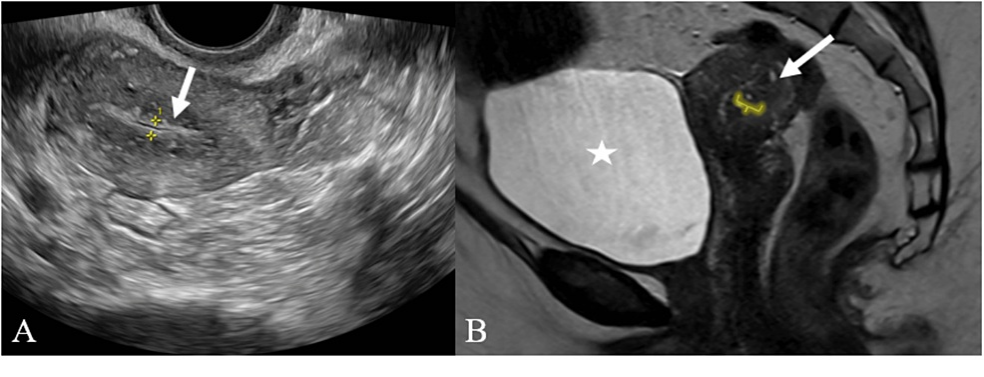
A case of adenomyosis A: USG of the pelvis longitudinal section showing sub-endometrial echogenic linear striations, extending from endometrium into inner myometrium (white arrow). Irregular endo-myometrial junction. Few hyperechoic islands were noted. B: Sagittal T2-weighted MRI of the pelvis showing widening of the junctional zone (yellow) and few cystic areas in anterior and posterior myometrium (white arrow). The urinary bladder is denoted by a star. USG - ultrasonography
